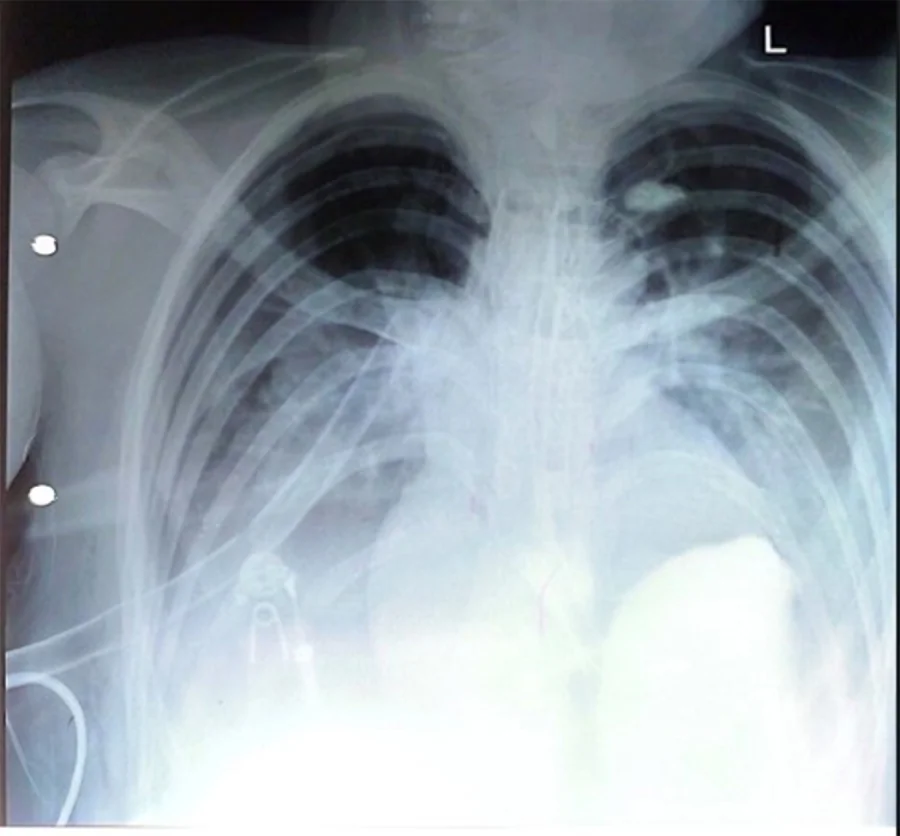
Post-chest tube insertion chest radiographs.
